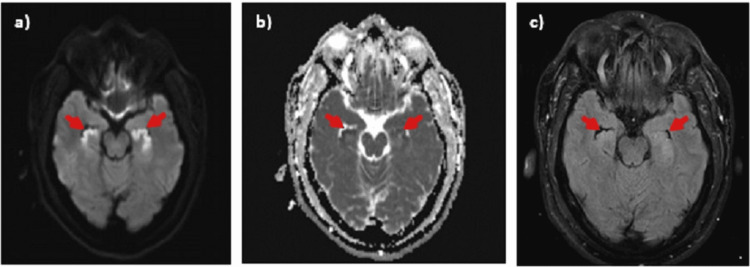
Brain MRI in Case 1, axial views. a) Diffusion-weighted imaging b) apparent diffusion coefficient sequences showing diffusion restriction with corresponding c) T2 fluid-attenuated inversion recovery hyperintensities in bilateral hippocampi (arrows).
